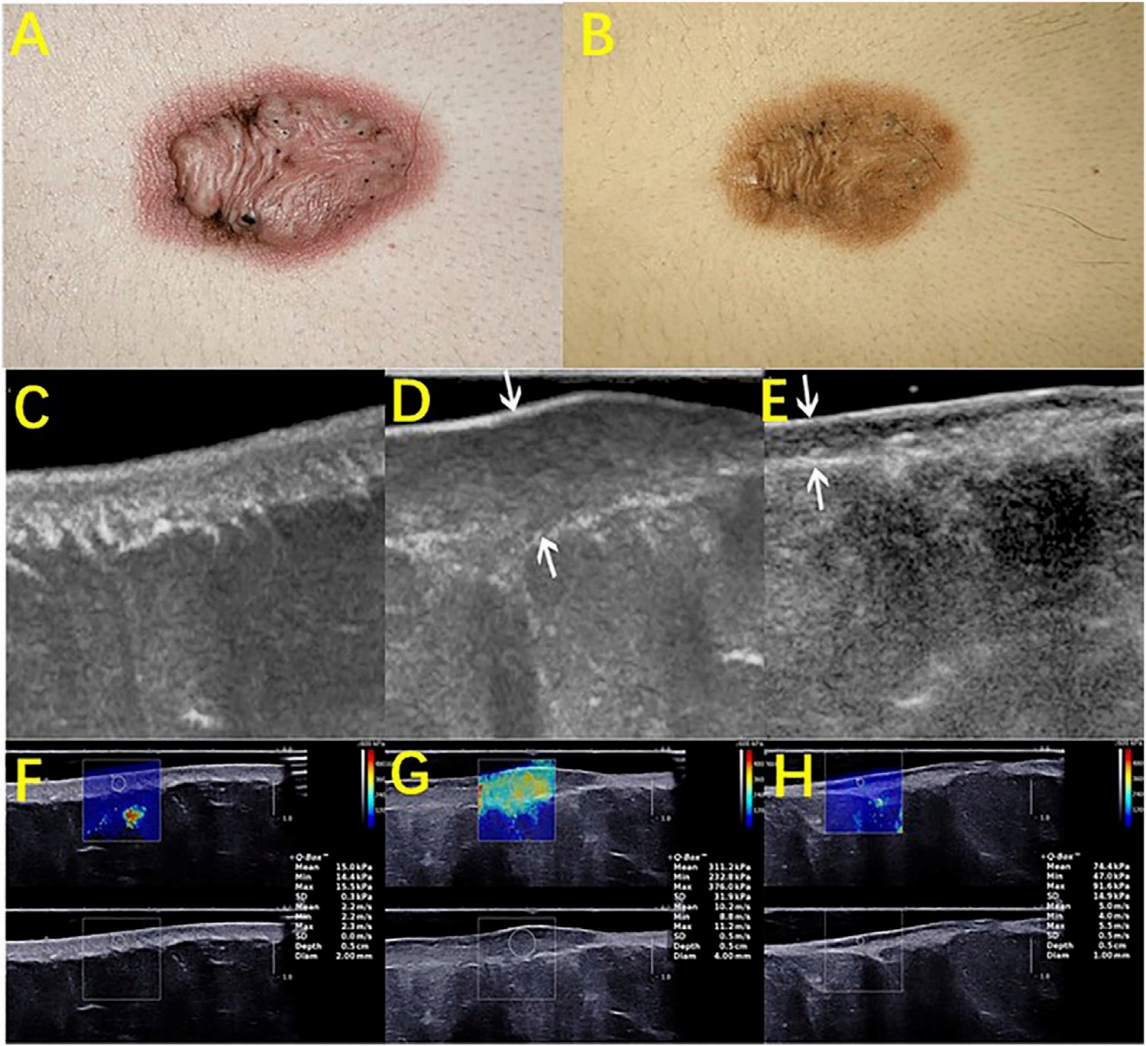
HFUS assessing the therapeutic efficacy of scars. A keloid before (A) and after (B) intralesional steroids injection. B-mode ultrasound images (longitudinal) of normal skin (C), pretreated keloids (D), and post treated keloids after an intralesional steroid injection (E). (F), (G), and (H) are the corresponding elastography images of (C), (D), and (E), respectively. Images (A) to (H) were captured from the same patient. Arrows in images (D) and (E) showed an obvious reduction in the scar thickness after treatment. The depth × width of the images (C–E) were 1.5 × 2.25, 1.6 × 2.4, and 1.5 × 2.25 (cm), respectively. As shown in the images, the quantitative elasticity values, including Young’s modulus and shear wave velocity, decreased after treatment.
